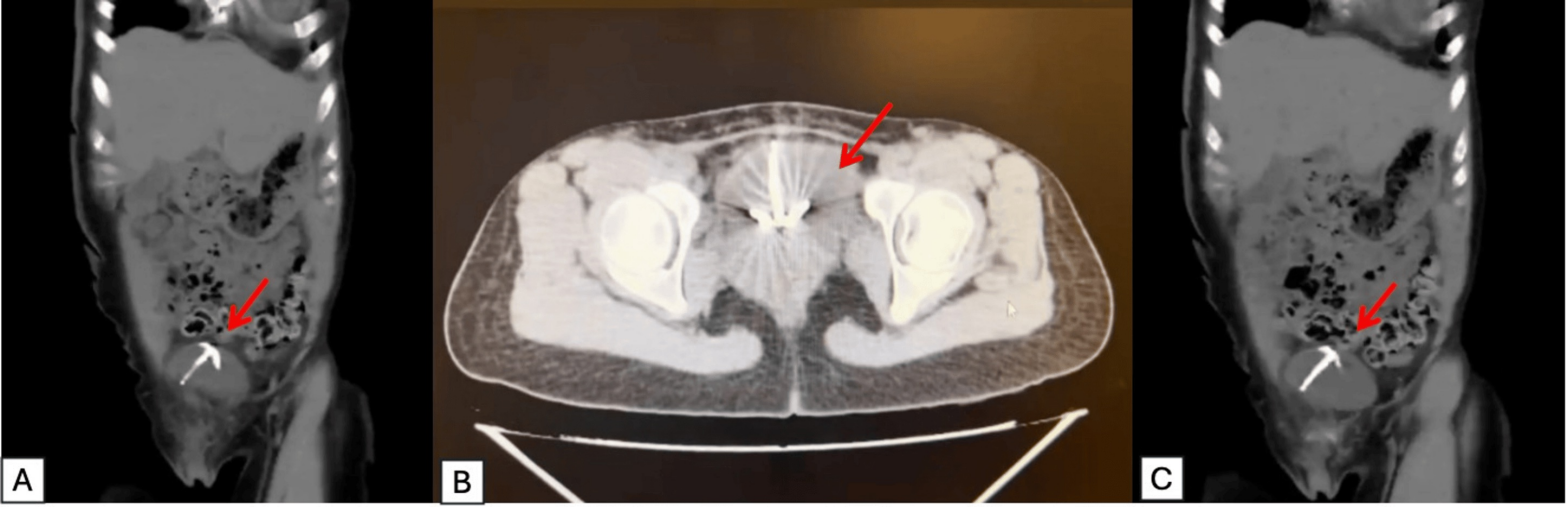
(A,C) CT noncontrast showing IUD with red arrow, coronal section. (B) Axial section CT: computed tomography; IUD: intrauterine device
